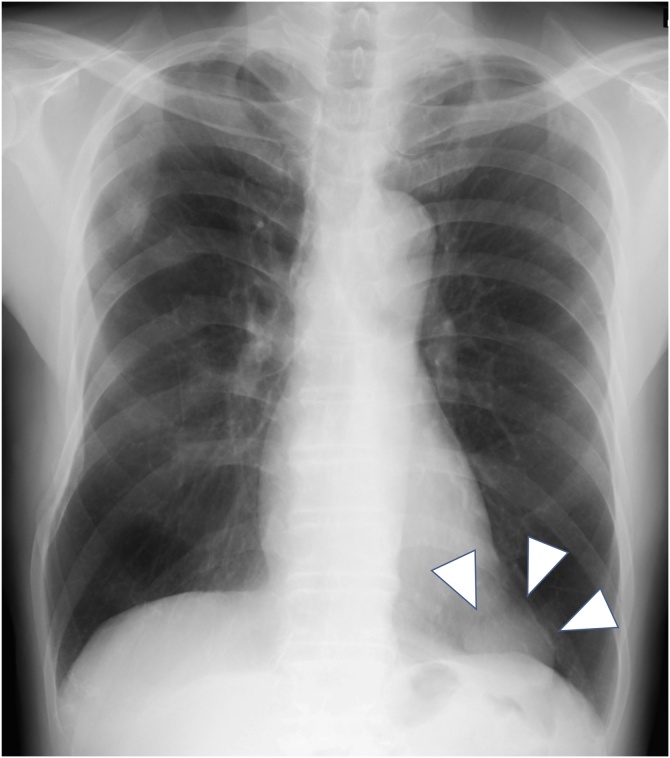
Chest X-ray showing a 50 mm-sized mass in the lower left lung field (arrowheads).
